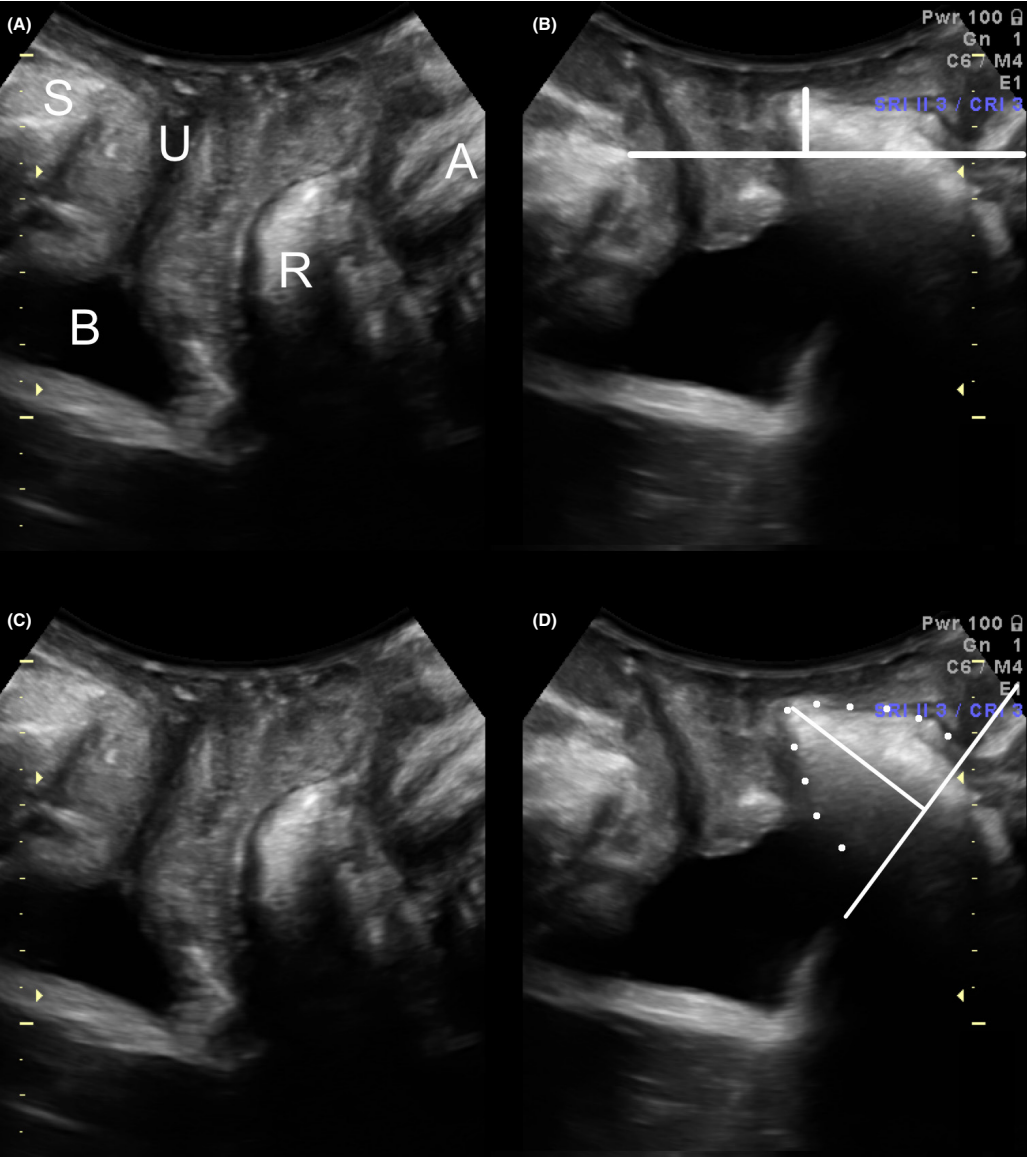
Prolapse assessment by translabial ultrasound, midsagittal plane. This is a typical small rectocele in a nulliparous patient. Panels A and C are at rest, panels B and D on Valsalva manoeuvre. The vertical line in Panel B shows descent of a small rectocele to about 1 cm below the symphyseal reference line. The oblique lines in Panel D show the base and depth of the rectocele which is outlined in dots. S, symphysis pubis; U, urethra; B, bladder; R, rectal ampulla; A, anal canal.
